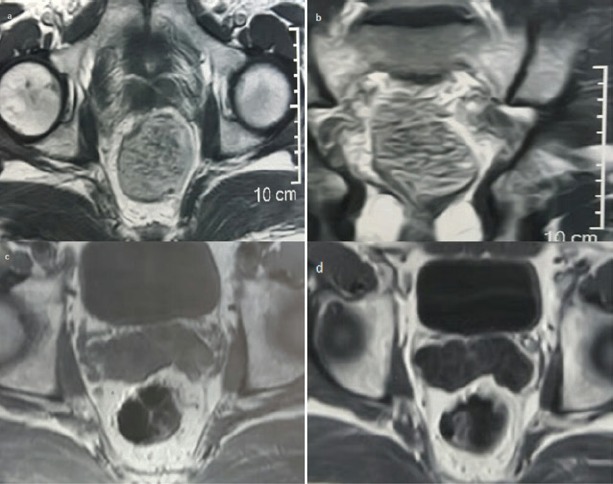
MRI of case one (a) before imatinib (axial section) (b) before imatinib (coronal section) (c) after 3 months imatinib (d) after 6 months imatinib.
